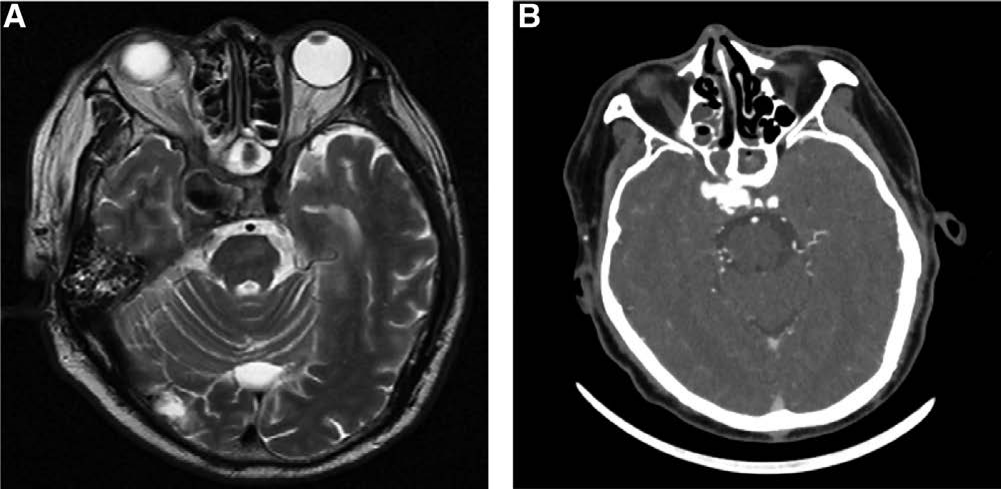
T2-weighted (A) MRI and CT angiogram (B) showing right internal carotid pseudoaneurysm measuring 20 × 15 mm. CT = computed tomography, MRI = magnetic resource imaging.
